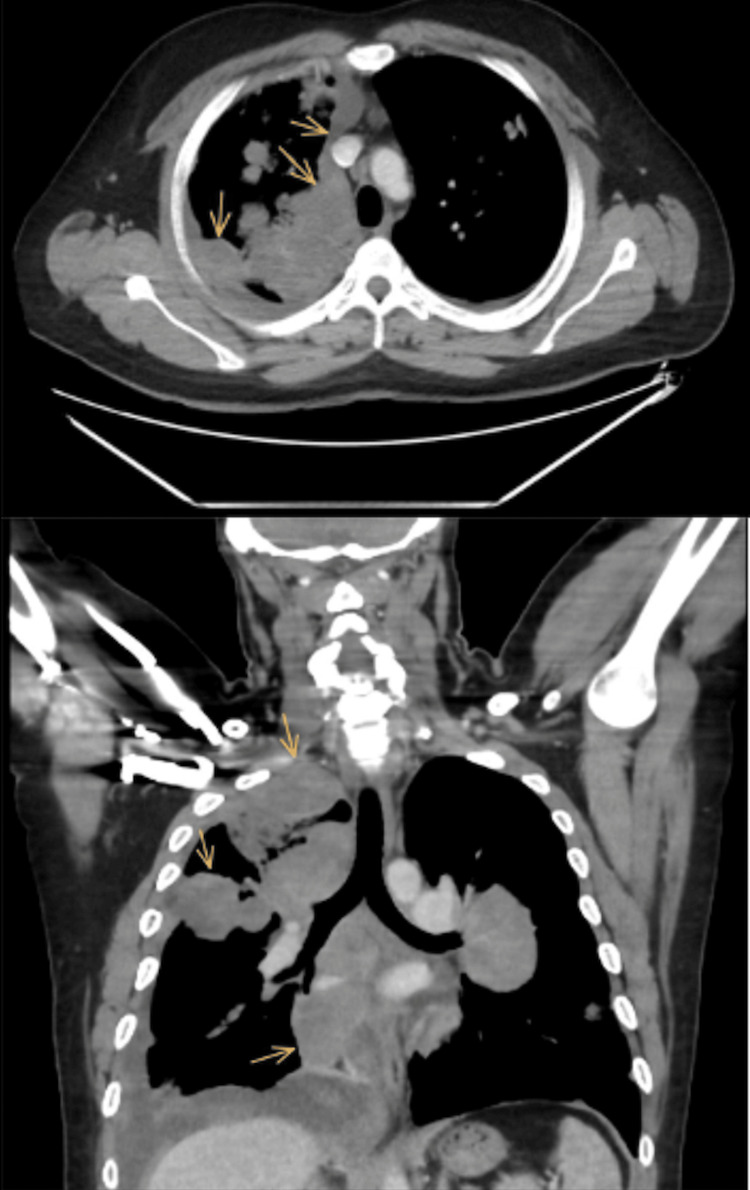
Axial and coronal CT chest with an IV contrast study showed pleural-based soft tissue pleural masses (yellow arrows) Computed tomography (CT); Intravenous (IV)
